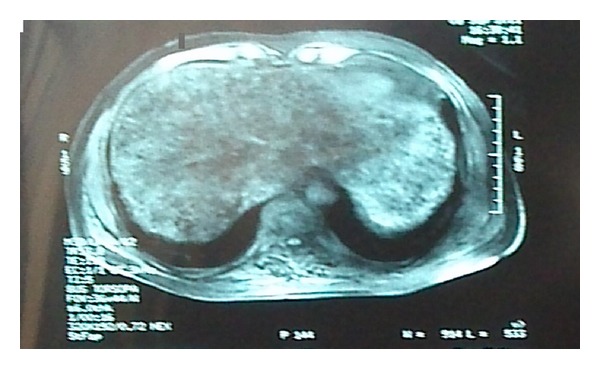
MRI depicting intrahepatic bile duct dilation.
